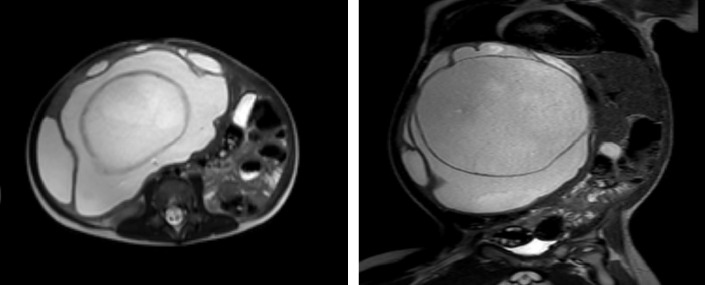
MRI abdomen showing a large 13.6 × 17.7 cm.
